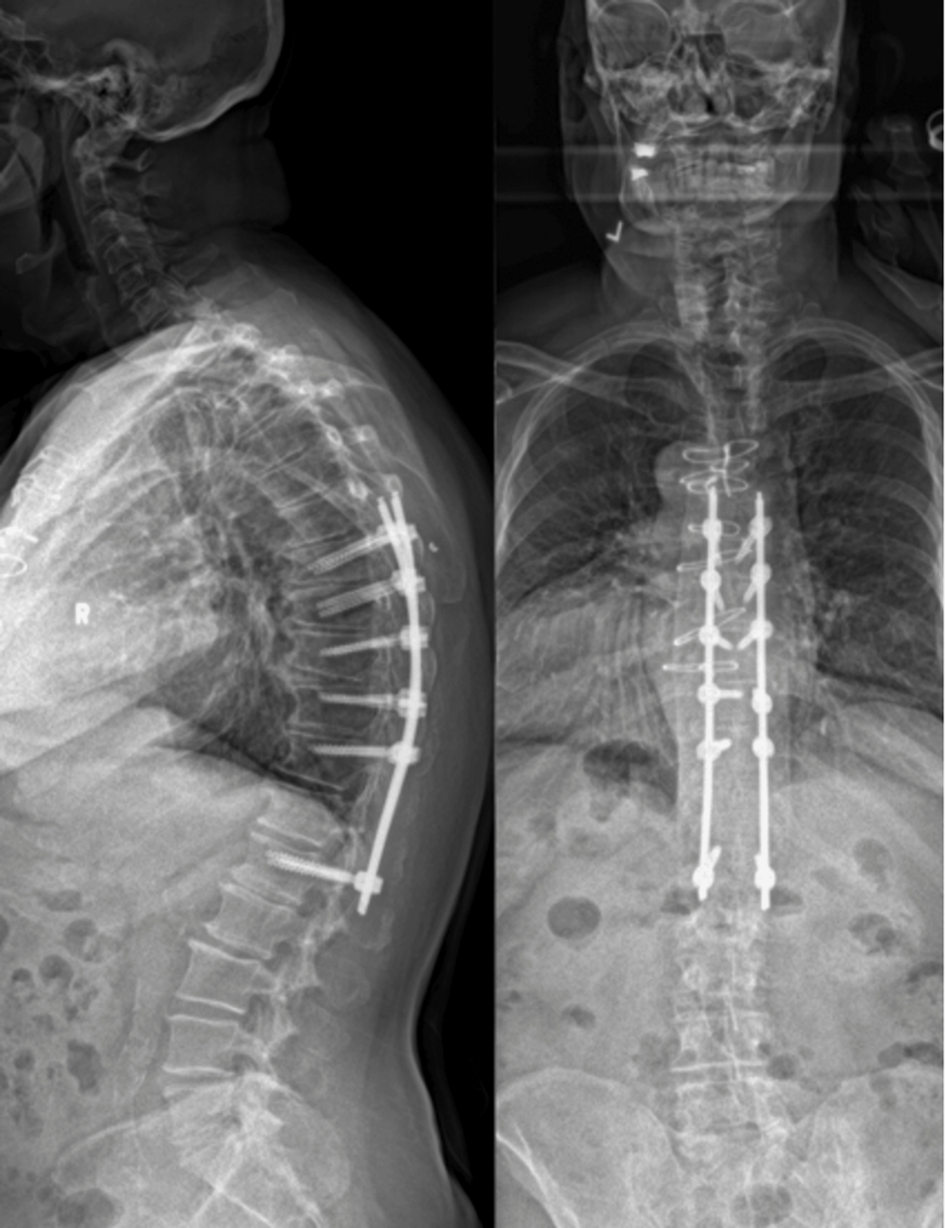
Postoperative AP/lateral radiographs at the sixth-month outpatient visit after undergoing MIS-PPS instrumentation. AP = anterior-posterior; MIS-PPS = minimally invasive stabilization-percutaneous pedicle screws
